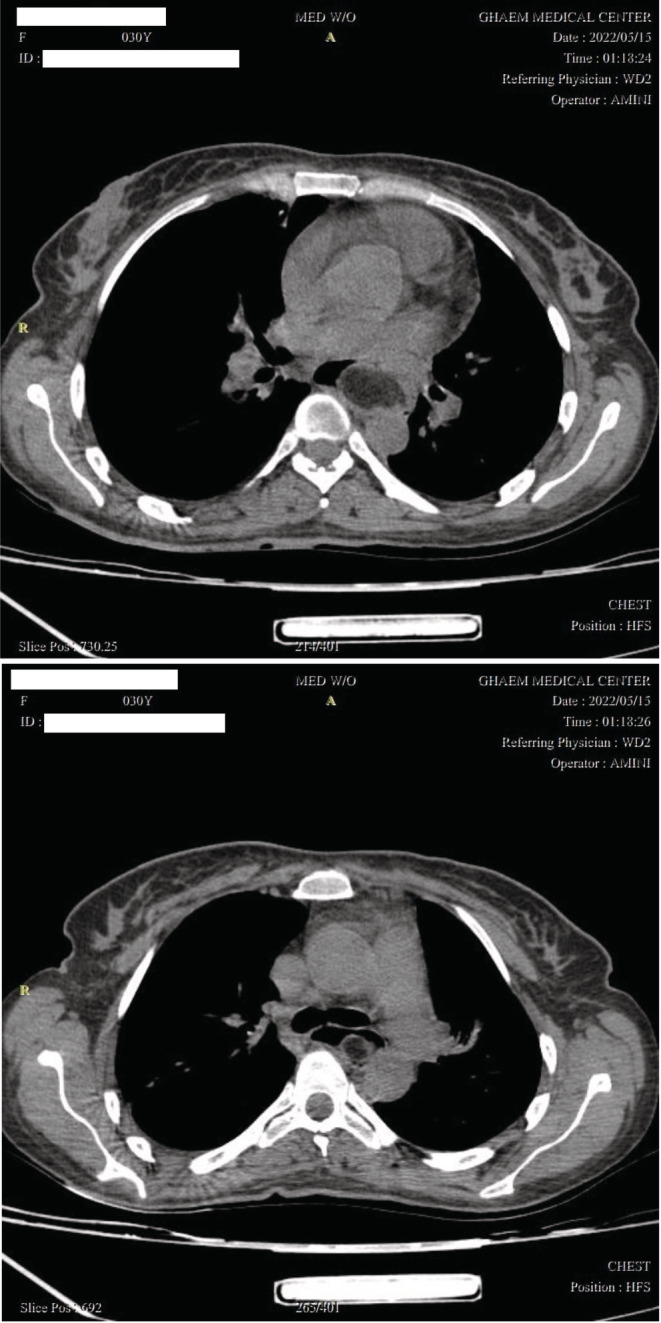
Axial views of the esophageal polyp CT scan
